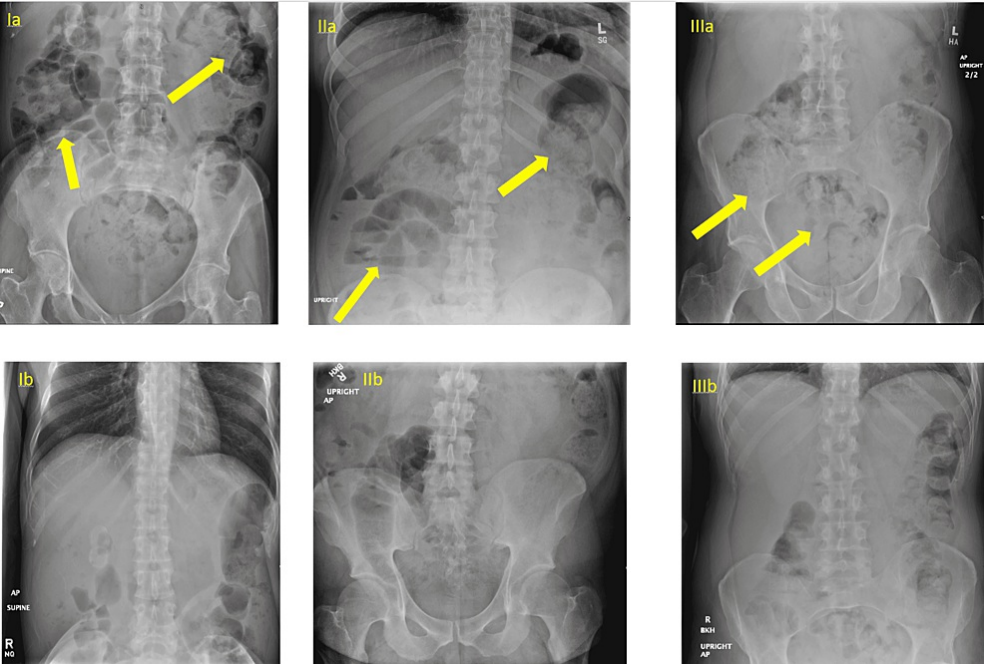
Abdominal X-rays of cases 1-3 Top row: Ia, IIa, and IIIa demonstrate abdominal X-rays of cases 1-3, respectively, before lubiprostone administration. Arrows indicate large areas of fecal stasis. Bottom row: Ib, IIb, and IIIb, demonstrate abdominal X-rays of cases 1-3, respectively, after lubiprostone administration.
